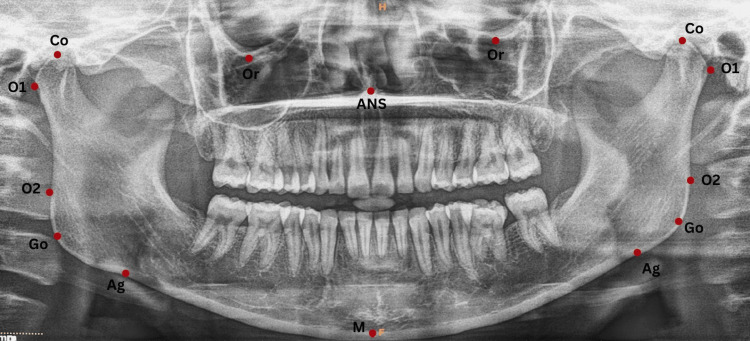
Landmarks identified on an orthopantomogram Or: Orbitale; Co: Condylion; Go: Gonion; Ag: Antegonion; M: midpoint on the inferior border of the mandible; ANS: anterior nasal spine; O1: the most lateral point on the condyle; O2: the most lateral point on the ascending ramus This figure represents the original orthopantomogram of the patient, obtained with the patient's permission, from the study.
